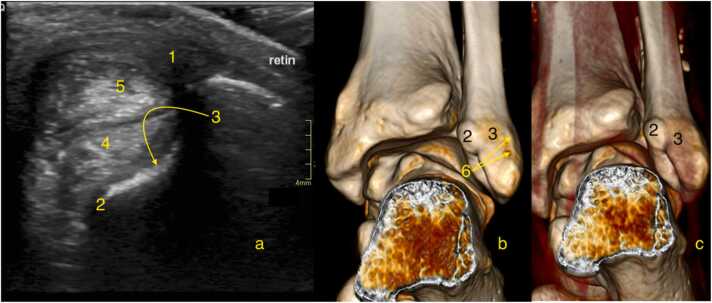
The retromalleolar groove. (A) Ultrasonography, (B, C) 3D reconstruction from computed tomography. 1- Superior peroneal retinaculum, 2- Retromalleolar tubercle, 3- Retromalleolar groove, 4- Peroneus brevis (tendon), 5- Peroneus longus, 6- Retromalleolar ridge. Retin - an abbreviation for the superior peroneal retinaculum. A subtle imprint of the peroneus tendons can be observed at the level of the posterior surface of the lateral malleolus, where the number 3 is visible, and slightly above it.
